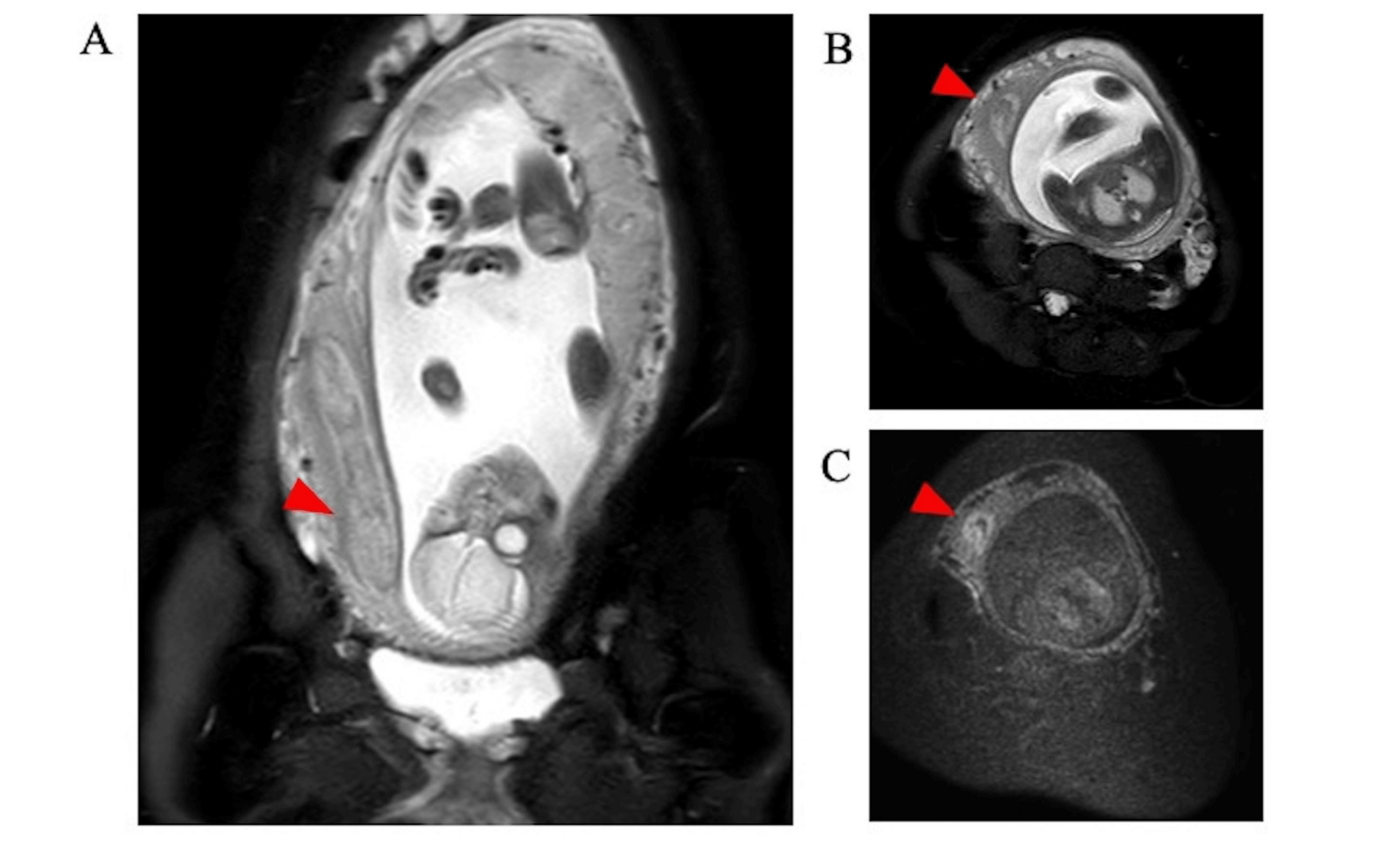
Magnetic resonance imaging of the abdomen. (A) T2-weighted image (coronal section). (B) T2-weighted image (horizonal section). (C) Diffusion-weighted image (horizonal section. The red arrow suggests a space-occupying lesion in the right uterus.
